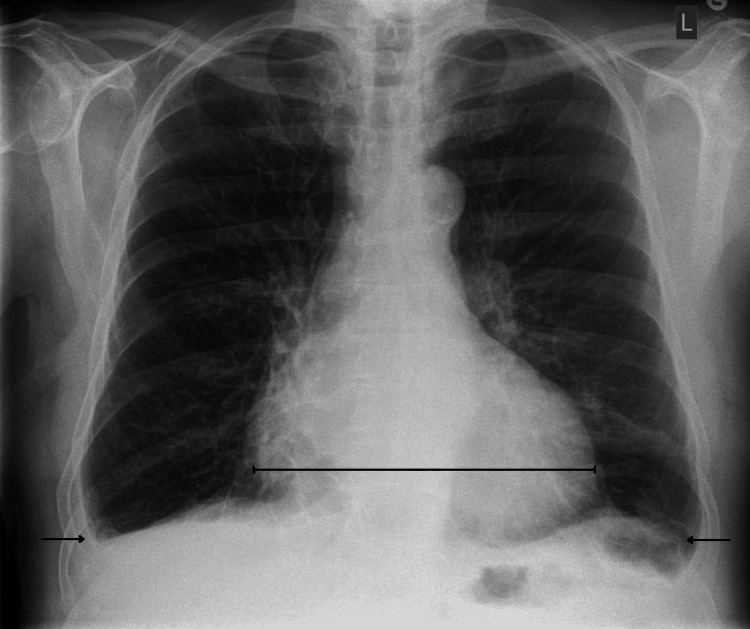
Chest X-ray Cardiomegaly (bracket line) with bilateral pleural effusions (arrows).
